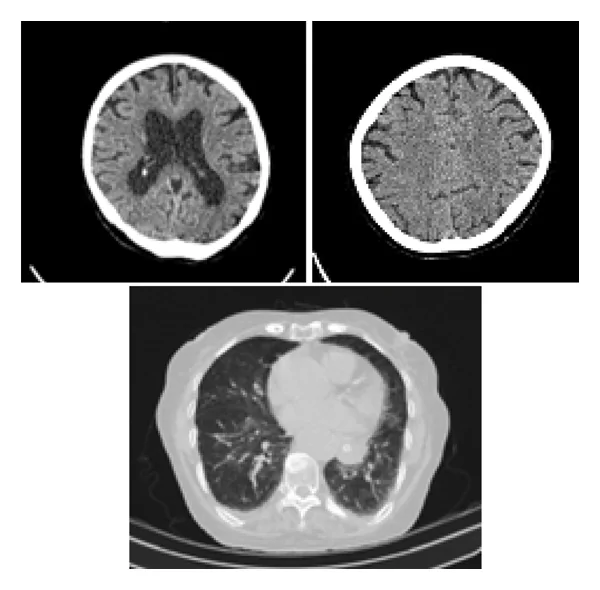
Brain CT scan showing no acute abnormalities, with no evidence of pulmonary embolism.
